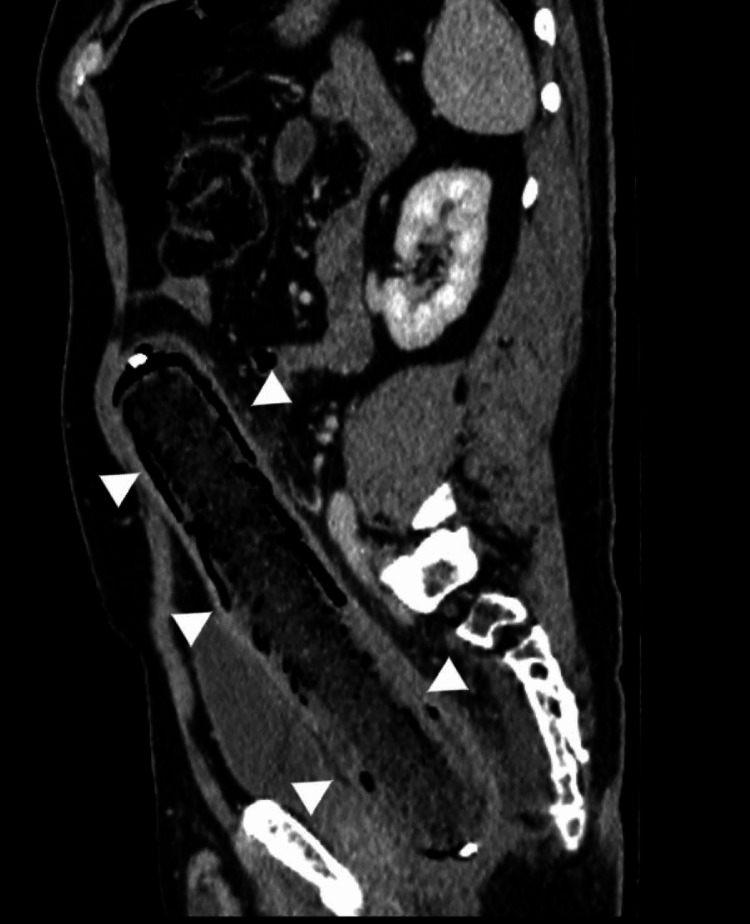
The oblique sagittal CT image reveals the presence of a baton sausage, which originates at the distal rectum and extends through the sigmoid colon (arrow heads). Note the bulging of the abdominal wall.
